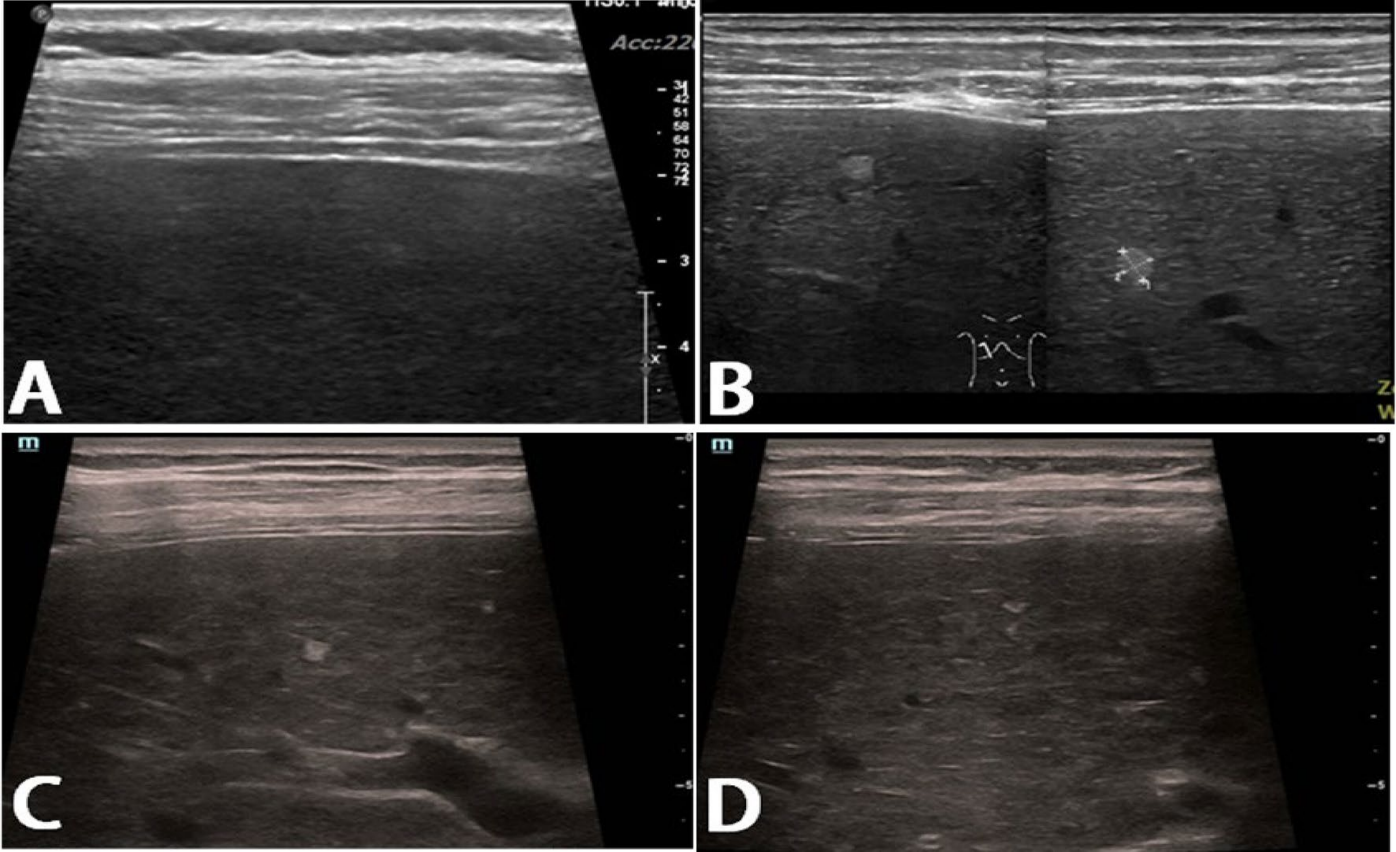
High-frequency ultrasound examination parameters and grading. (A) No nodule in liver (grade 0), fine granular echo in liver parenchyma (grade 0); (B) Scattered small hyperechoic nodules were seen in the liver, and the echo of the liver parenchyma showed coarse granules (grade 0); (C, D) The hyperechoic structure of the liver parenchyma was widened and lengthened, with an irregular shape and cord-like shape. The hypoechoic structure between the cords was irregular and widened, with strong and hypoechoic structures arranged in a staggered manner, showing a large cribriform or reticular shape. In the reticular shape, multiple hypoechoic nodules (grade 1) were scattered in the liver parenchyma.
